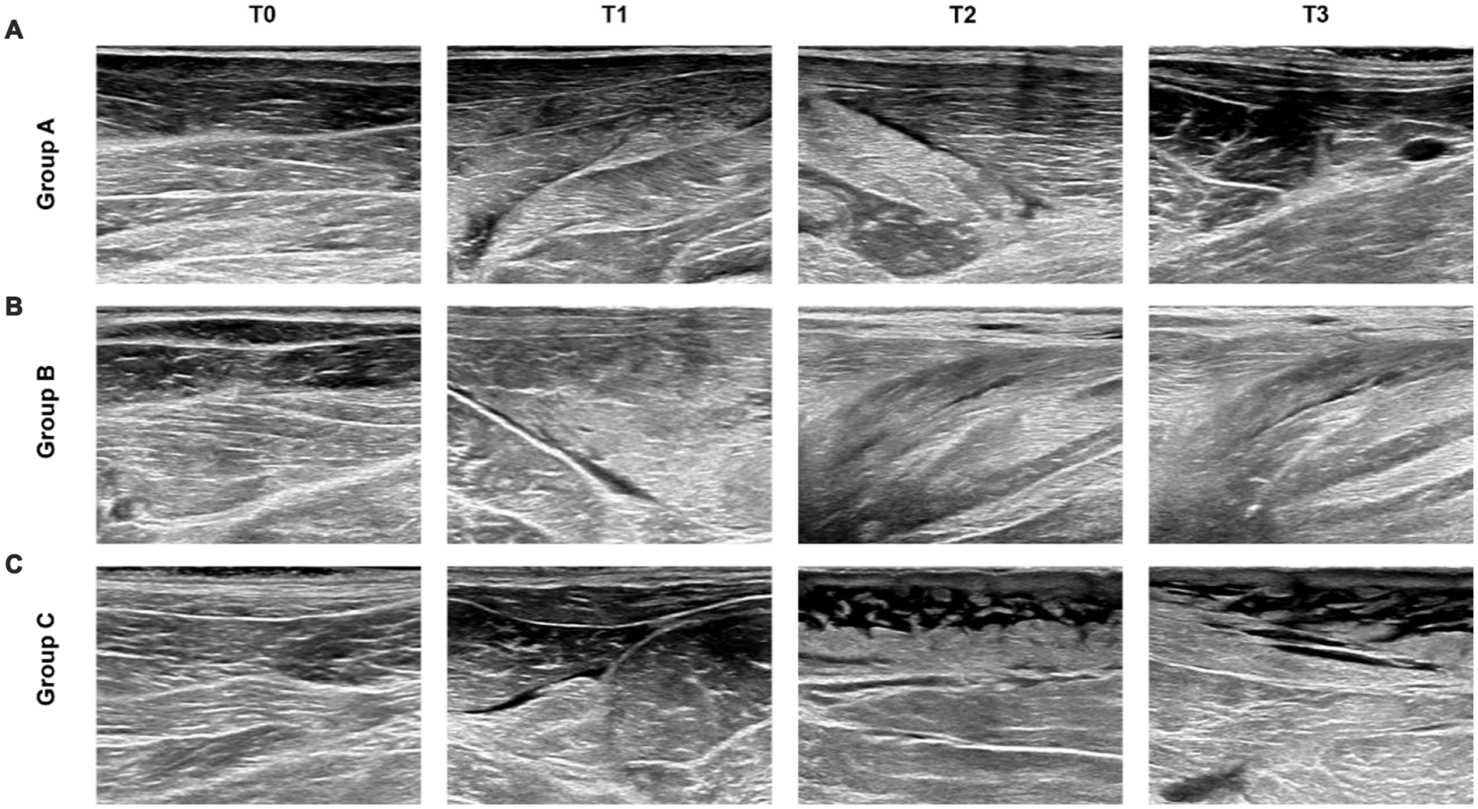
2D ultrasound findings in groups A, B, and C post-decompression. (a) Group A: Mild echogenicity enhancement with preserved texture, indicating gradual recovery. (b) Group B: Muscle thickening with blurred texture, suggesting partial recovery. (c) Group C: Disordered echogenicity (“sieve-like” hypoechoic, “cloudy” appearance), showing progressive worsening.
